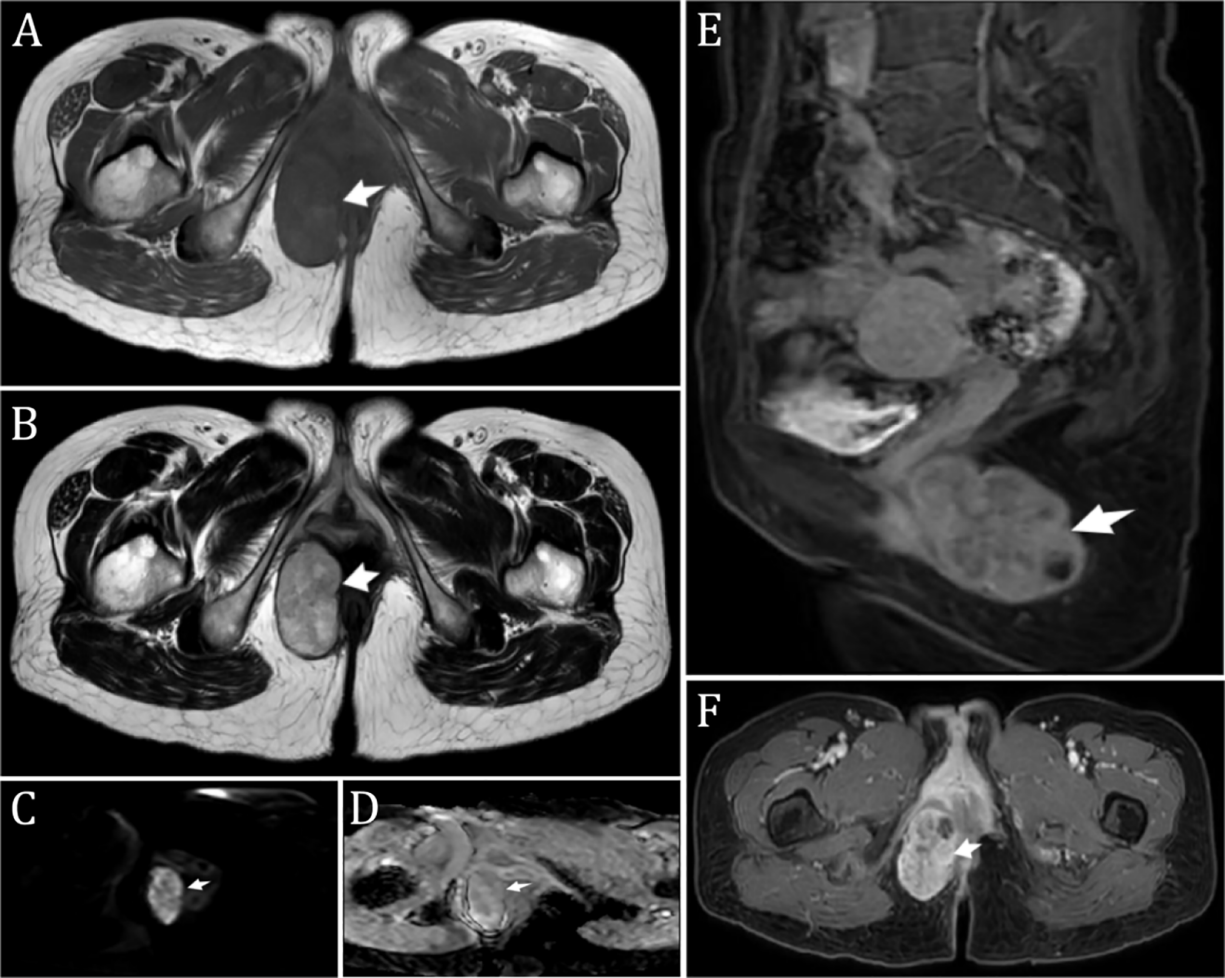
Preoperative Imaging (A, B). T1-weighted image (A) and T2-weighted image (B) showing a perianal mass in the right gluteal subcutaneous fat. The mass exhibited iso- to slightly hyperintense T1 and T2 signals, with a clear boundary and measuring approximately 5.8 cm × 3.3 cm × 5.0 cm; (C) Diffusion-weighted imaging (DWI) displaying high signal intensity; (D) Apparent diffusion coefficient (ADC) showing iso-to-low signal intensity; (E) Sagittal fat-suppressed contrast-enhanced T1 axial image; (F) Enhanced image showing uneven, significant enhancement with patchy areas of liquefactive necrosis within. *The arrow indicates the mass (considering malignancy).
